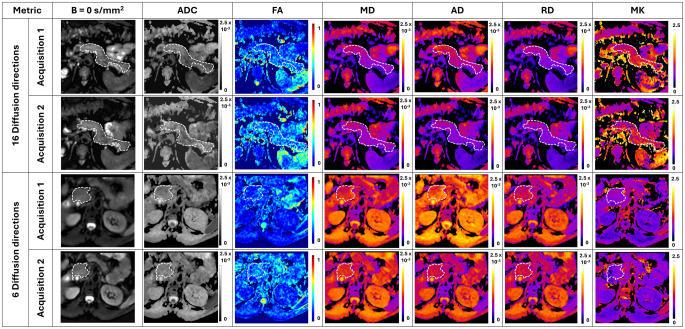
DWI, DTI and DKI maps in two volunteers, for each MRI protocol (6 vs. 16 diffusion directions). Examples show both acquisitions for each volunteer. The pancreas is delimited in white in both volunteers, showing mostly homogeneous values in all maps, with a similar visual quality as the clinical standard ADC maps. MD, AD, RD and ADC maps are expressed in mm^2^/s
